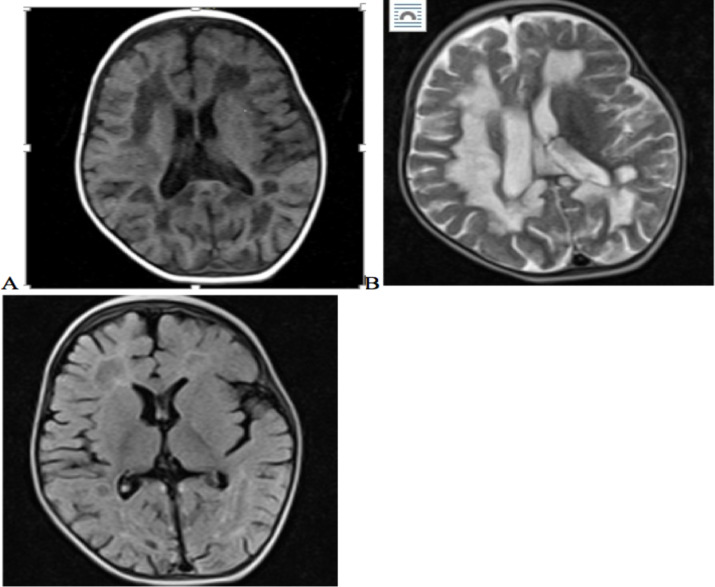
Pre-contrast axial T1-weighted magnetic resonance imaging (A) showing diffuse white matter hypo intensity involving the centrum semiovale and corona radiata. Axial T2-weighted magnetic resonance imaging (B) showed diffuse hyper intensity. Corpus callosum involvement was seen as hyper intensity; mainly in the splenium. U fibers are spared. Fluid attenuated inversion recovery (FLAIR) images (C) showed hypo intense areas, which have signal intensity values close to that of cerebrospinal fluid and were interspersed within the white matter. Basal ganglia, internal capsules, and external capsules were spared.
